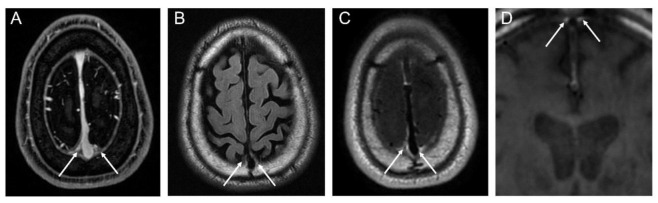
MRI visualization of peri-sinus lymphatic space on conventional contrast-enhanced axial T1-weighted image (A), axial FLAIR image (B), contrast-enhanced axial T1-weighted black-blood image (C), and coronal T1-weighted black-blood image (D).
